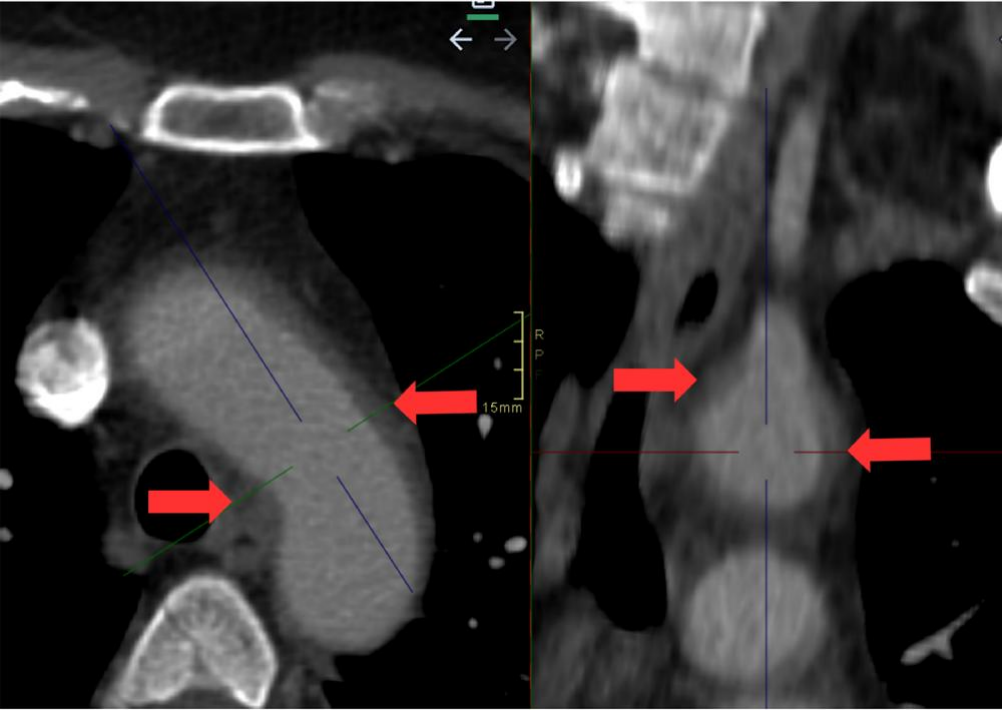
Contrast computed tomography: arrows demonstrated irregular wall thickening of the ascending and arch of the aorta with periaortic fat stranding.
